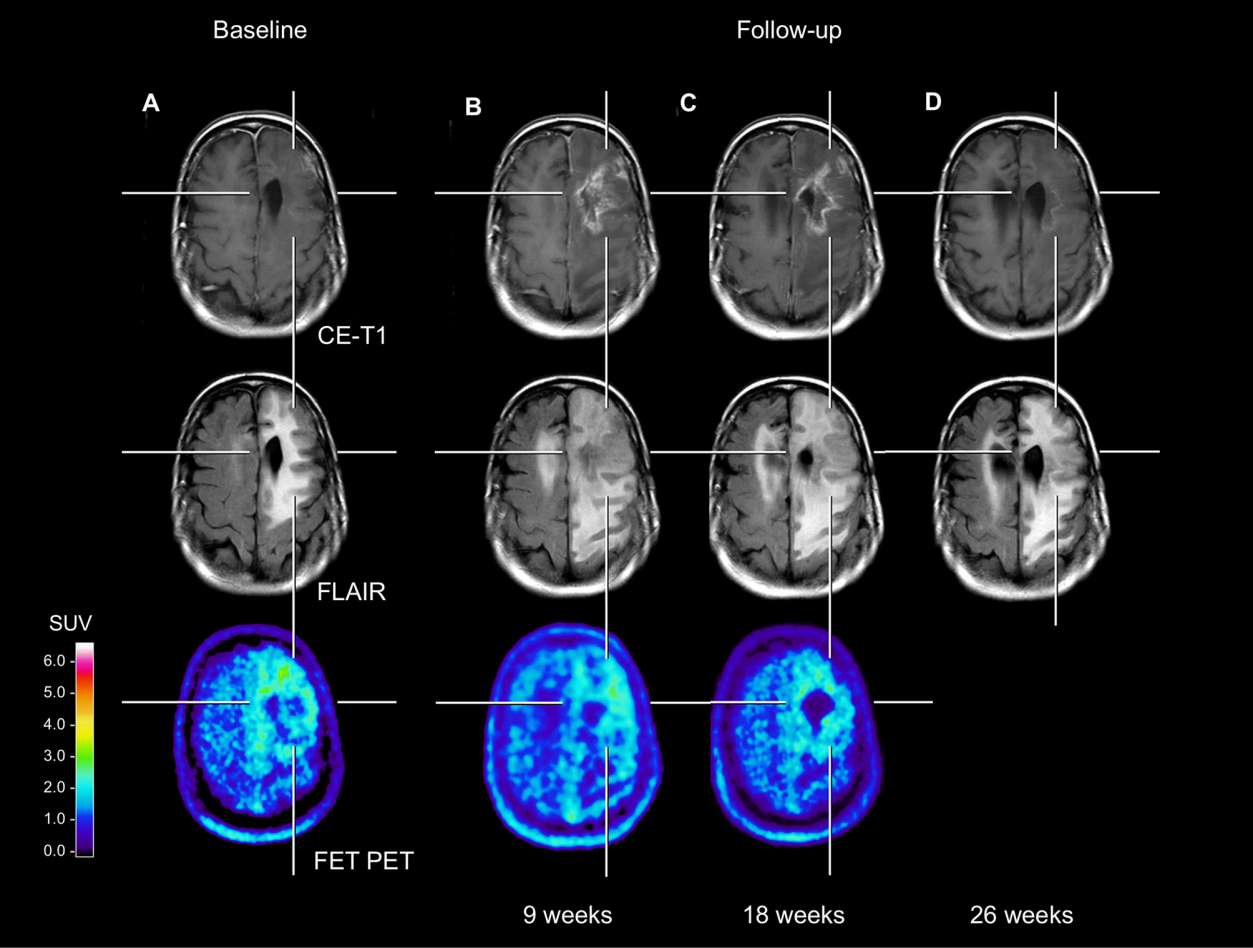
Neuroimages including contrast-enhanced MRI, FLAIR-weighted MRI, and FET PET of a patient with an anaplastic oligodendroglioma (IDH mutant, 1p/19q co-deleted). Compared to the baseline MRI (A), the follow-up MRI perfomed 9 weeks later (17 weeks after end of radiotherapy) (B) showed a substantial contrast enhancement and an extensive FLAIR signal hyperintensity in the area of the resection defect. In contrast, both the baseline and first follow-up FET PET (A, B) showed no pathologically increased tracer uptake (mean tumor-to-brain ratios in both scans, 1.8). The rationale for this is based on the fact that several FET PET studies suggested that especially in pretreated glioma patients a mean tumor-to-brain ratio of 2.0 separates best between tumor relapse and treatment-related changes (1, 6, 8). The second follow-up MRI (C) showed a further slight increase of the FLAIR signal alteration. Again, the corresponding follow-up FET PET showed no substantial change of metabolic activity compared to the previous examinations (A, B). Not earlier than 26 weeks, the third follow-up MRI (D) revealed an almost complete decrease of the contrast enhancement.
